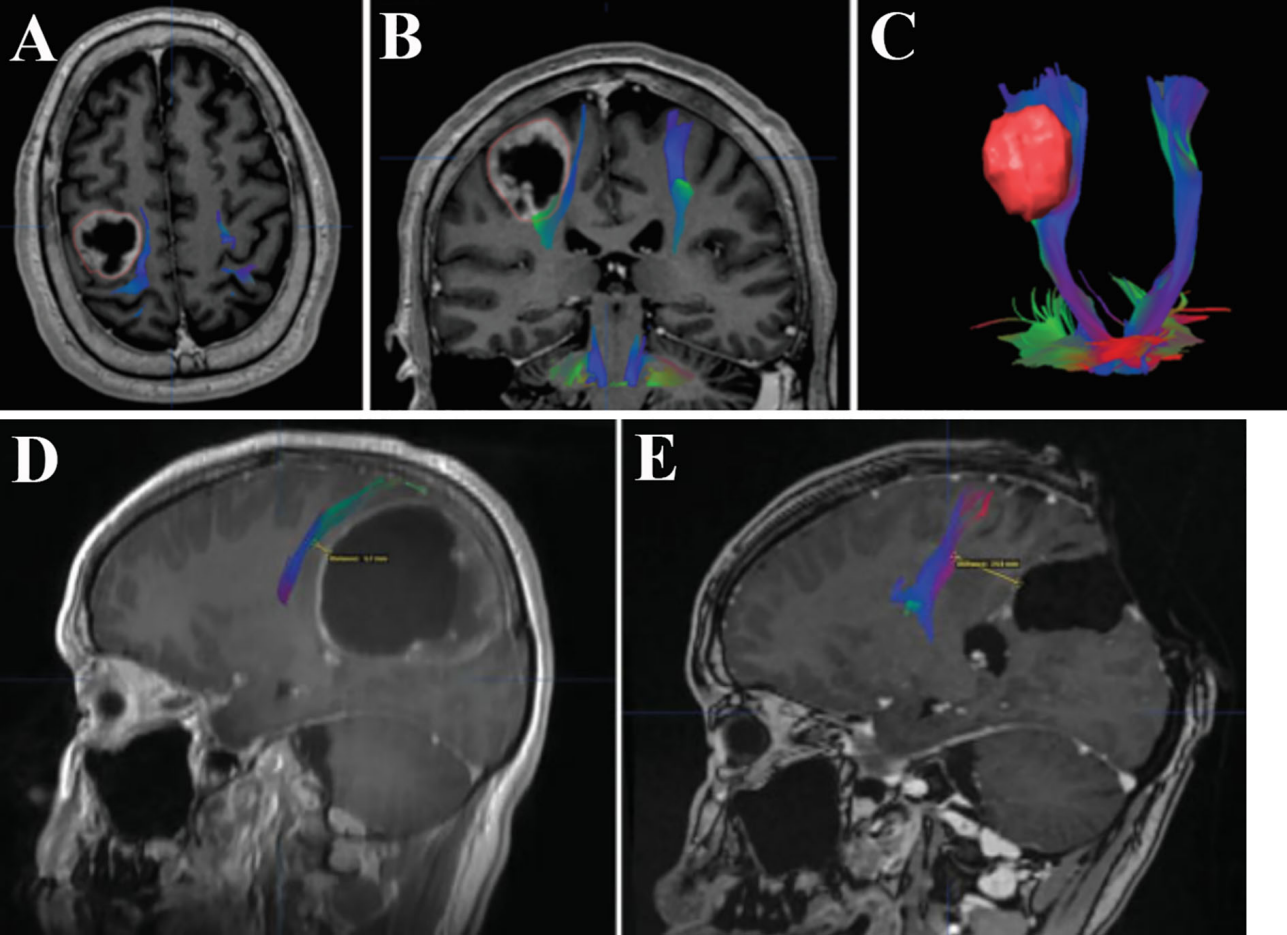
Merged DTI sequence and contrast T1-weighted MRI revealing the proximity between BM and the CST. A cystic lesion with peripheral contrast enhancement allocated in the perirolandic region was depicted in the axial (A) and coronal (B) planes. The proximity was noted between the deep surface of the tumor and the descending fibers of the CST (B). The 3D reconstruction of the CST and volumetric reconstruction of the lesion showed the distortion of the motor pathways (C). Total removal of another large cystic tumor which significantly displaced the CST (D) was confirmed by postoperative MRI (E). Notably, the distance between surgical cavity and the CST increased from 5.7 to 24.9 mm, suggesting the effect of initial deformation accompanied by intraoperative brain shift. DTI, diffusion tensor imaging; MRI, magnetic resonance imaging; BM, bran metastases; CST, corticospinal tract. (A–C) Modified from Sanmillan et al. (4). (D, E) Modified from Krivosheya et al. (60).
